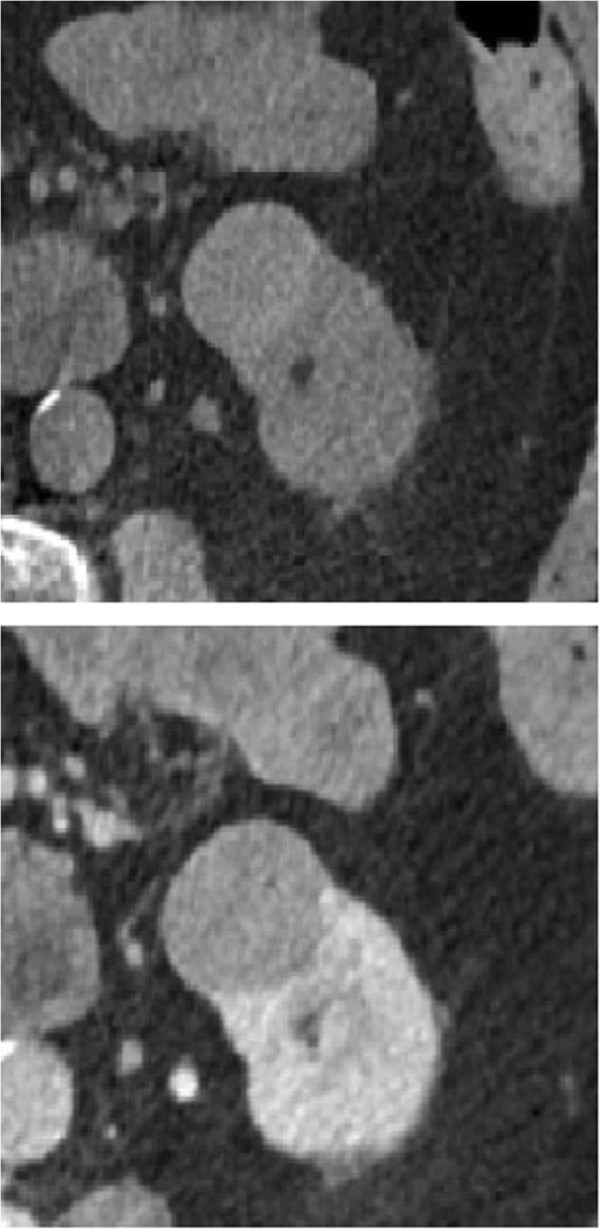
Radiological imaging of the patient's kidney tumor (a) (top): Unenhanced CT of kidney and tumor.
